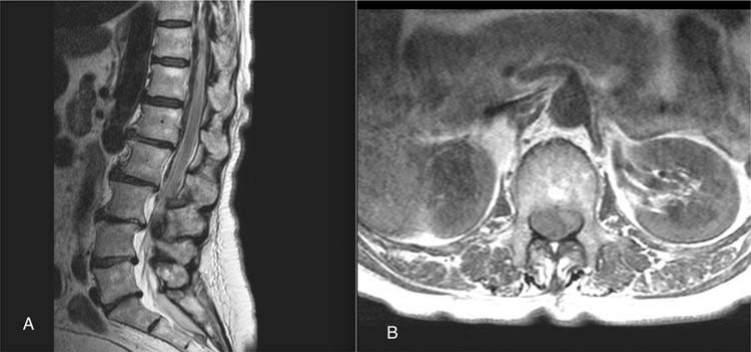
(A) The sagittal T2 FSE MRI show hypointense spindle-like mass from T10 to L3; (B) axial T1w FSE MRI showed iso-hyperintense hematoma compressing the dural sac (L1 level).
